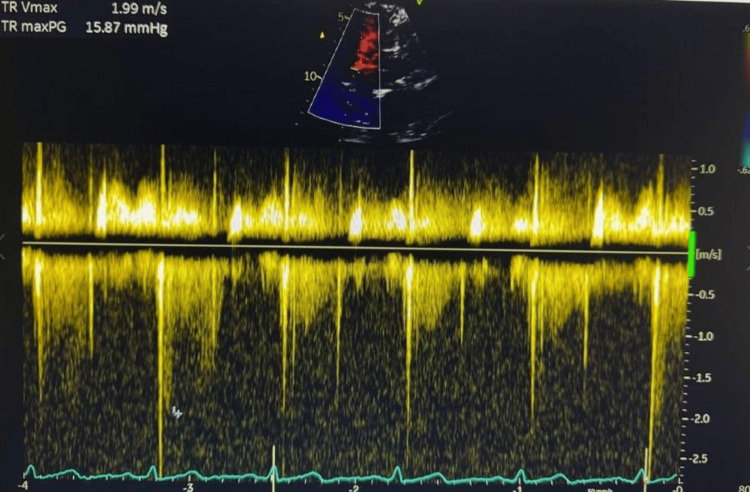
Preoperative continuous-wave Doppler showing tricuspid regurgitation with a peak velocity of 1.99 m/s and pressure gradient of 15.87 mmHg, indicating normal pulmonary artery pressure.
